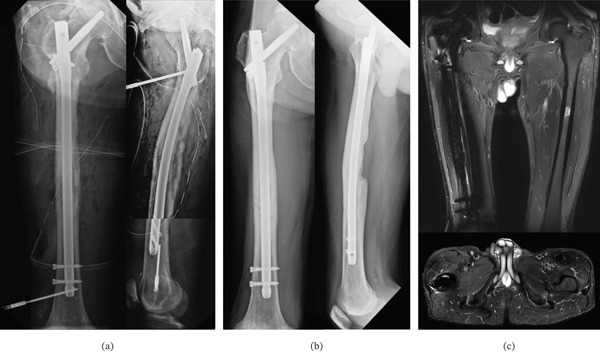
Case 2. (a) Anteroposterior and lateral radiographs of the right hip and femur after internal fixation with an intramedullary femoral nail combined with continuous local antibiotic perfusion (CLAP), (b) anteroposterior and lateral radiographs of the right hip and femur taken 6 months after internal fixation with CLAP, and (c) coronal and axial MRI with STIR images of bilateral hips and femurs taken 6 months after internal fixation plus CLAP.
